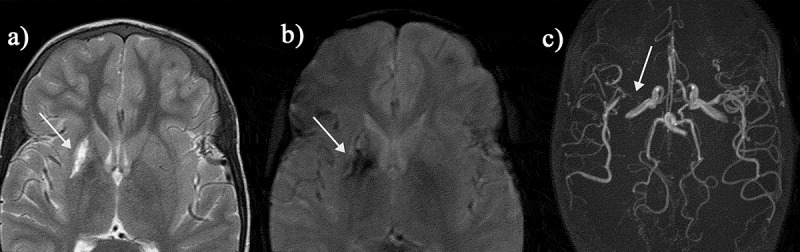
(a) Hemorrhagic basal ganglia infarct in a 16-yr-old girl not reporting symptoms but with asymmetric transcranial Doppler velocities (right time averaged mean of the maximum 39, left 164 cm/sec). The subtle hemorrhagic change is seen as a dark blush on the T2-weighted sequence but is well seen on the (B) T2* sequence (arrows). (C) A magnetic resonance angiogram revealed severe stenosis of the right middle cerebral artery with reduced filling of the distal vessels (geen arrow). She was followed for 3 years and did not develop neurological symptoms
